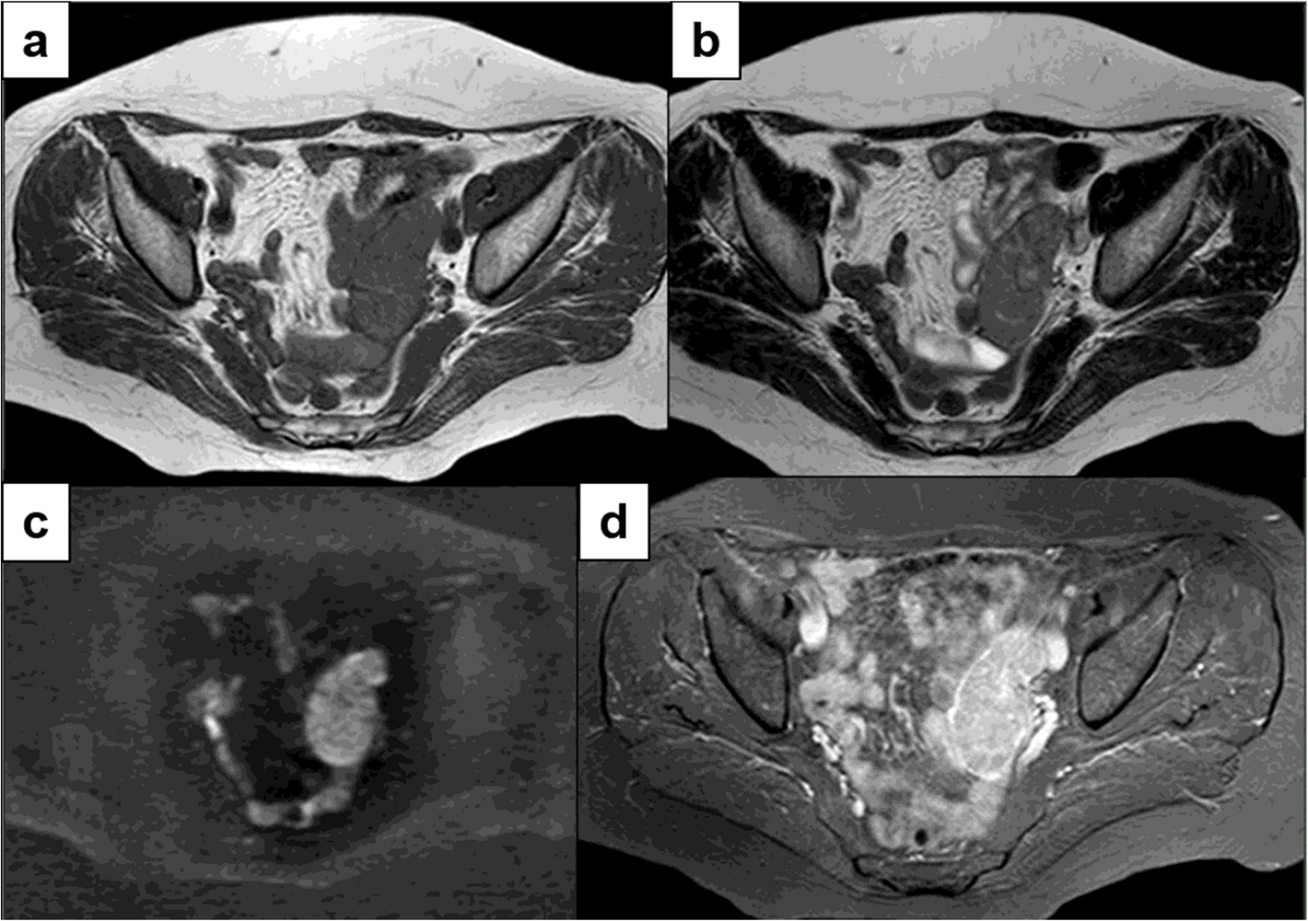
Ovarian dysgerminoma in a 34-year-old woman. Axial T1w (a), axial T2w (b), axial DWI (c), axial contrast-enhanced T1w with fat-suppression (d). MR images show an ovoidal, lobulated left ovarian mass, isointense on T1w, with heterogeneous predominantly hyper-intense signal on T2w, a restricted diffusion signal on DWI and an avid enhancement after Gd administration, with interlobular septa
